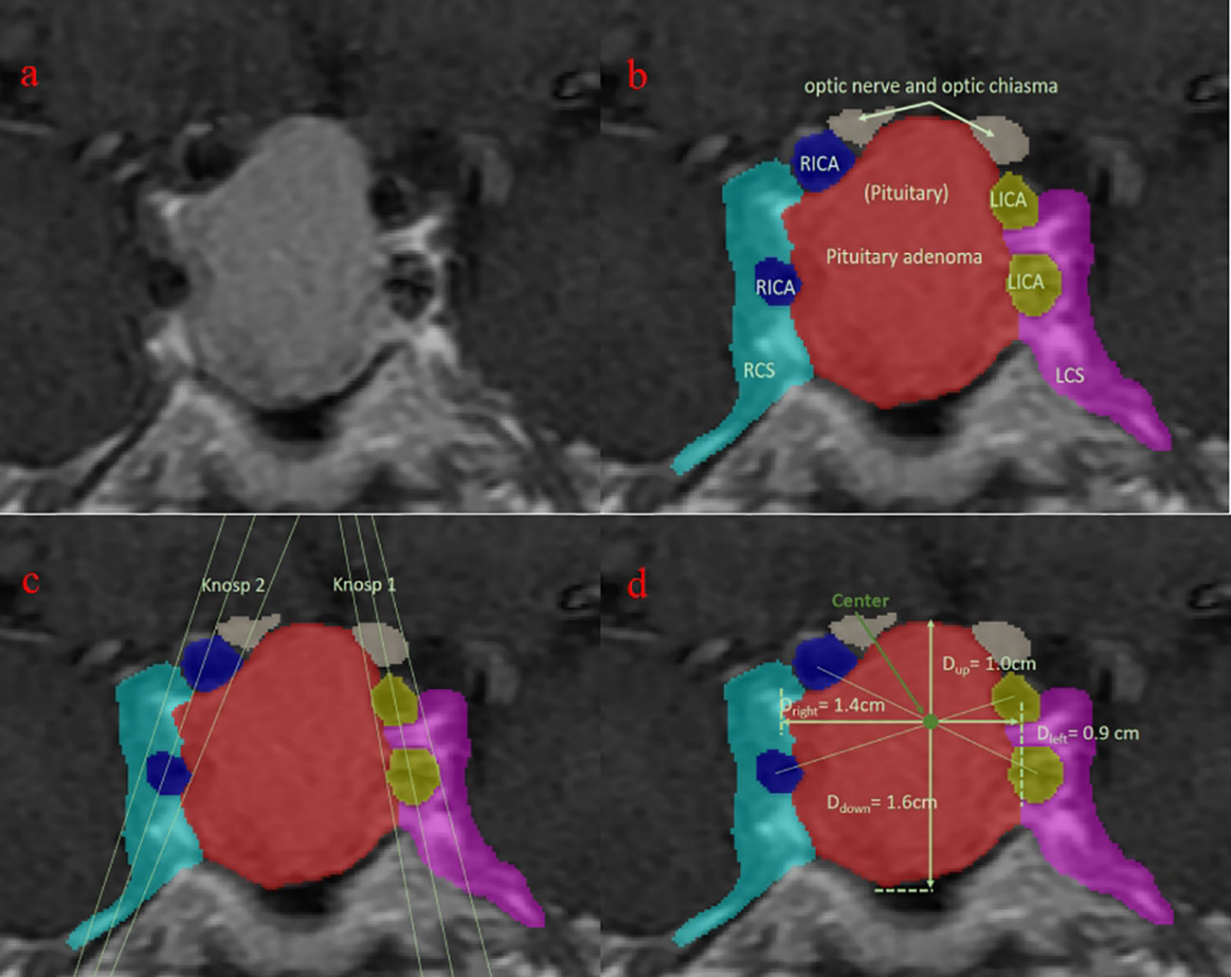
Visualization of the segmentation. Blue, right internal carotid artery; green: normal pituitary (not seen); light blue: right cavernous sinus; purple, left cavernous sinus; red: pituitary adenoma; white, optic nerve and optic chiasm; yellow, left internal carotid artery. (A) original MRI slice with the largest tumor area; (B) segmentation of different tissues; (C) Knosp grade based on the segmentation; (D) upward growth distance of pituitary adenoma.
